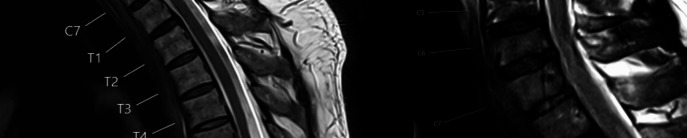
A Preoperative T2 sagittal MRI of 58-year-old male, depicting a SAC on levels T5-7. He presented with back pain and hyperreflexia and was surgically treated with a complete resection. Postoperatively the pain subsided, and he showed no new neurological deficits. B Preoperative T2 sagittal MRI of a 54-year-old male, depicting a SAW on level T3 with a proximal syrinx. He presented with radiating pain in his right arm, paresthesia of the right arm and was treated with a full resection. Postoperatively the pain subsided. The paresthesia was reduced but still present upon discharge and completely resolved upon last follow-up
